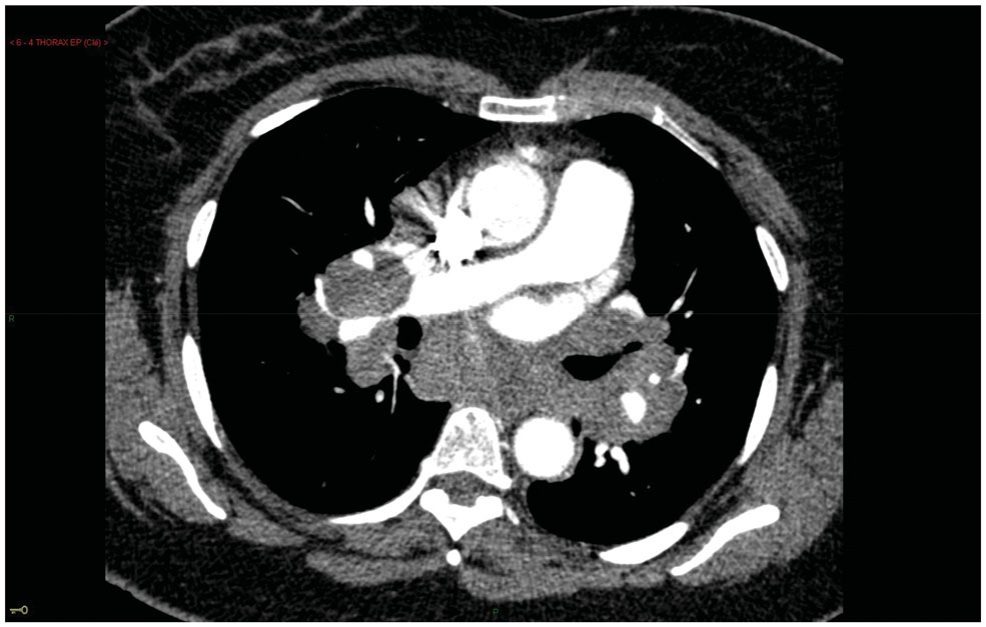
SAPH due to extrinsic vascular compression. CT scan shows compression of the right mediastinal pulmonary artery by enlarged lymph nodes. Note the increased diameter of main pulmonary artery as compared to aorta diameter.
